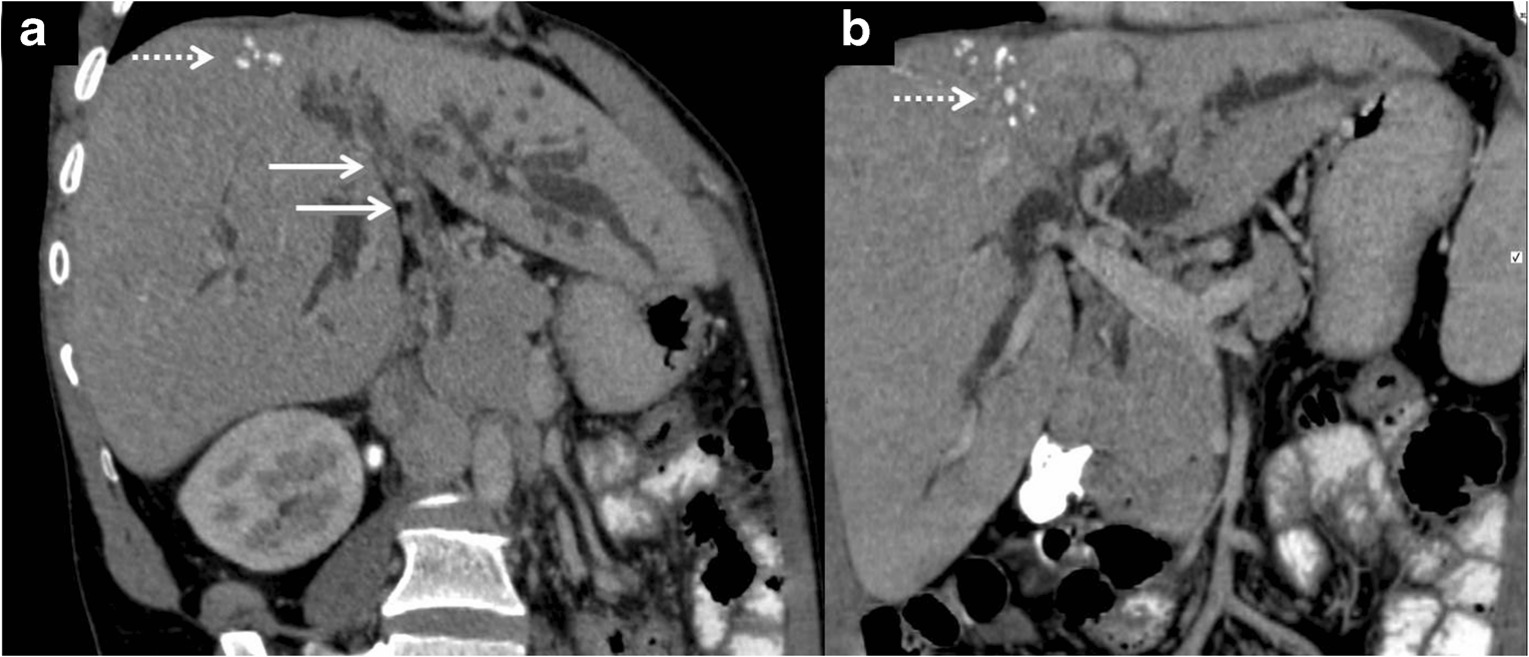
Coronal-oblique and coronal CT images in a patient with tubercular cholangitis displaying thickening and stricture of the extrahepatic common duct (*arrows*) with upstream intrahepatic biliary dilatation. The presence of hepatic calcifications (*dotted arrows*) help exclude cholangiocarcinoma and instead consider tuberculosis in appropriate clinical settings
